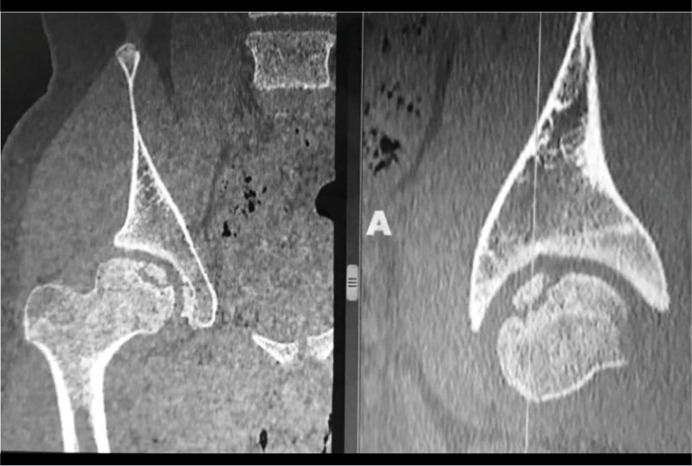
Computed tomography (CT) scan in the coronal (left) and axial (right) views. Note the elevation of the osteochondral (OCD) fragment in both views. There is no visible bone connection between the OCD fragment and the remaining of the femoral head.
